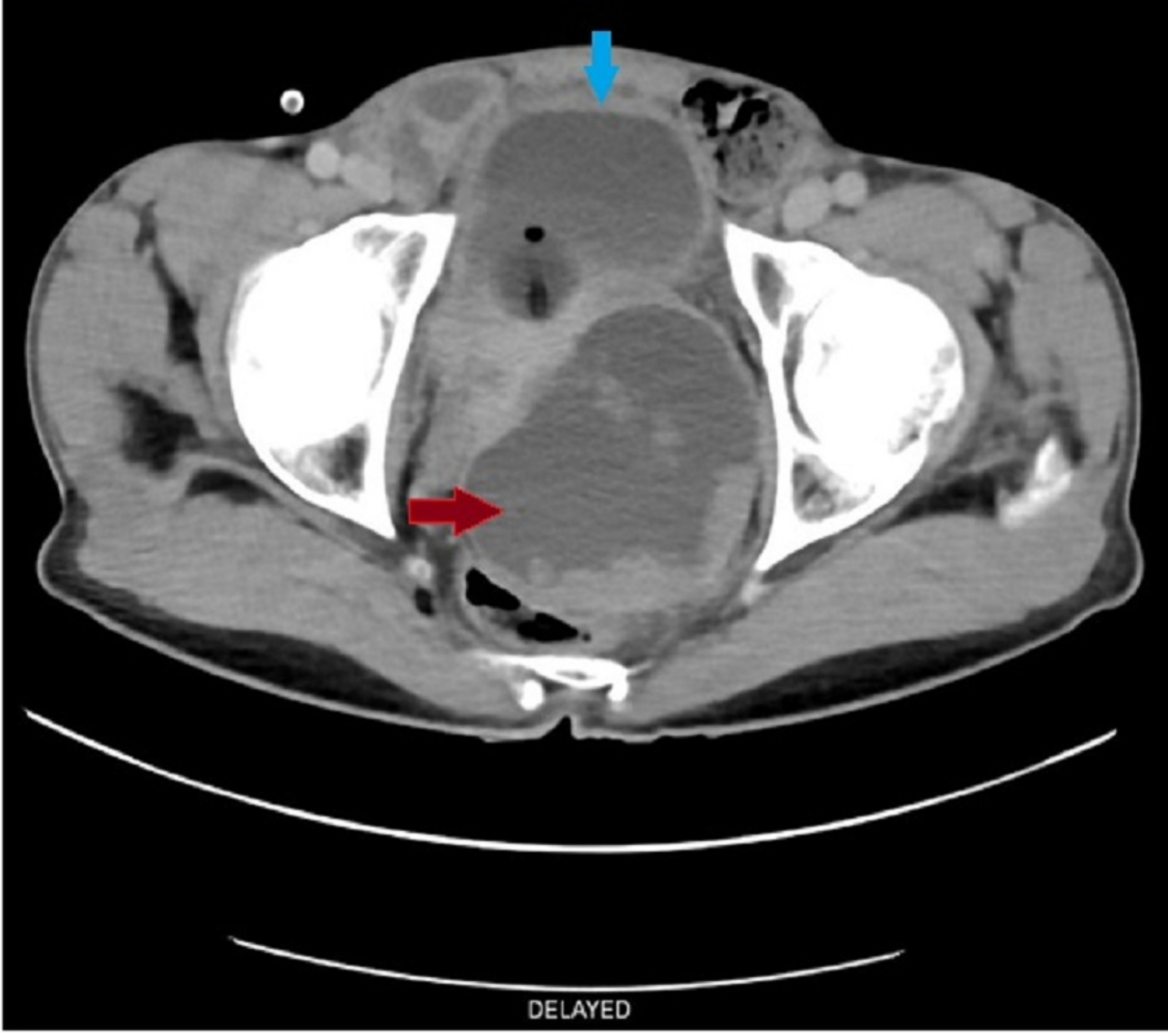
Contrast-enhanced computed tomography with axial image delayed phase showing mid circumferential wall thickening of the urinary bladder with Foley’s bulb (blue arrow) and prostatic cyst (red arrow)
